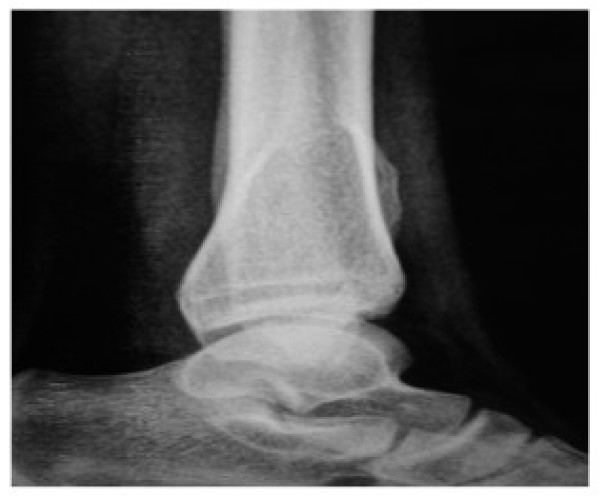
Lateral Radiograph of ankle of a patient showing well defined exostosis arising from interosseous border of tibia pushing fibula.
